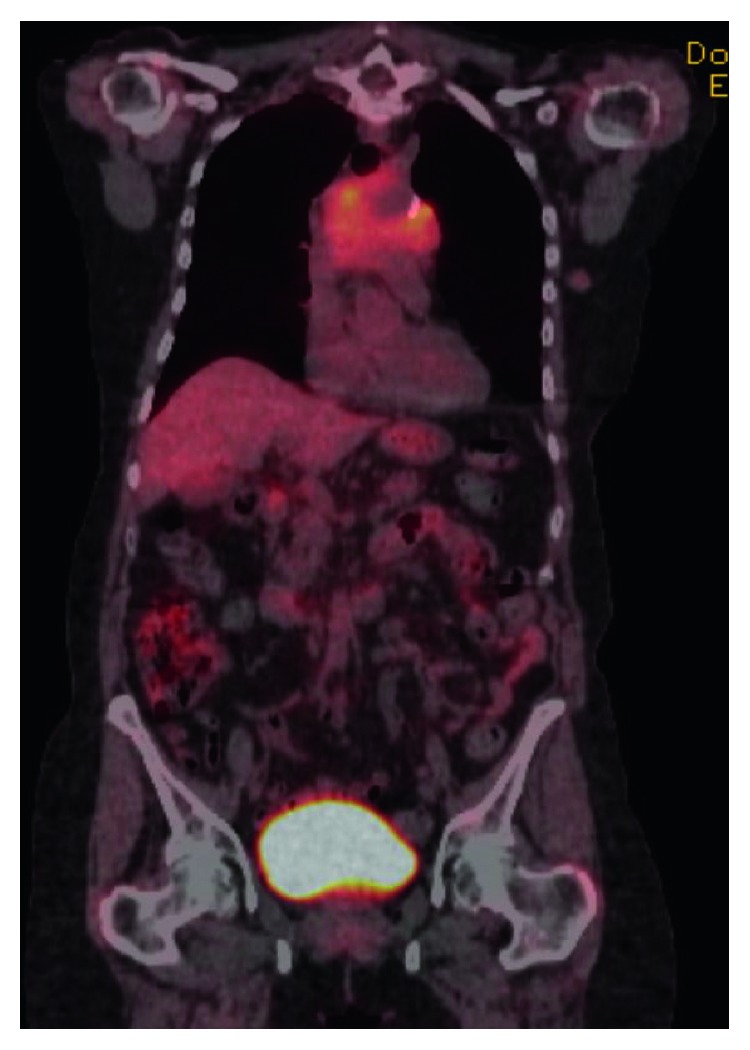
PET/CT with an infiltrative mass in the mediastinum with diffuse uptake.
